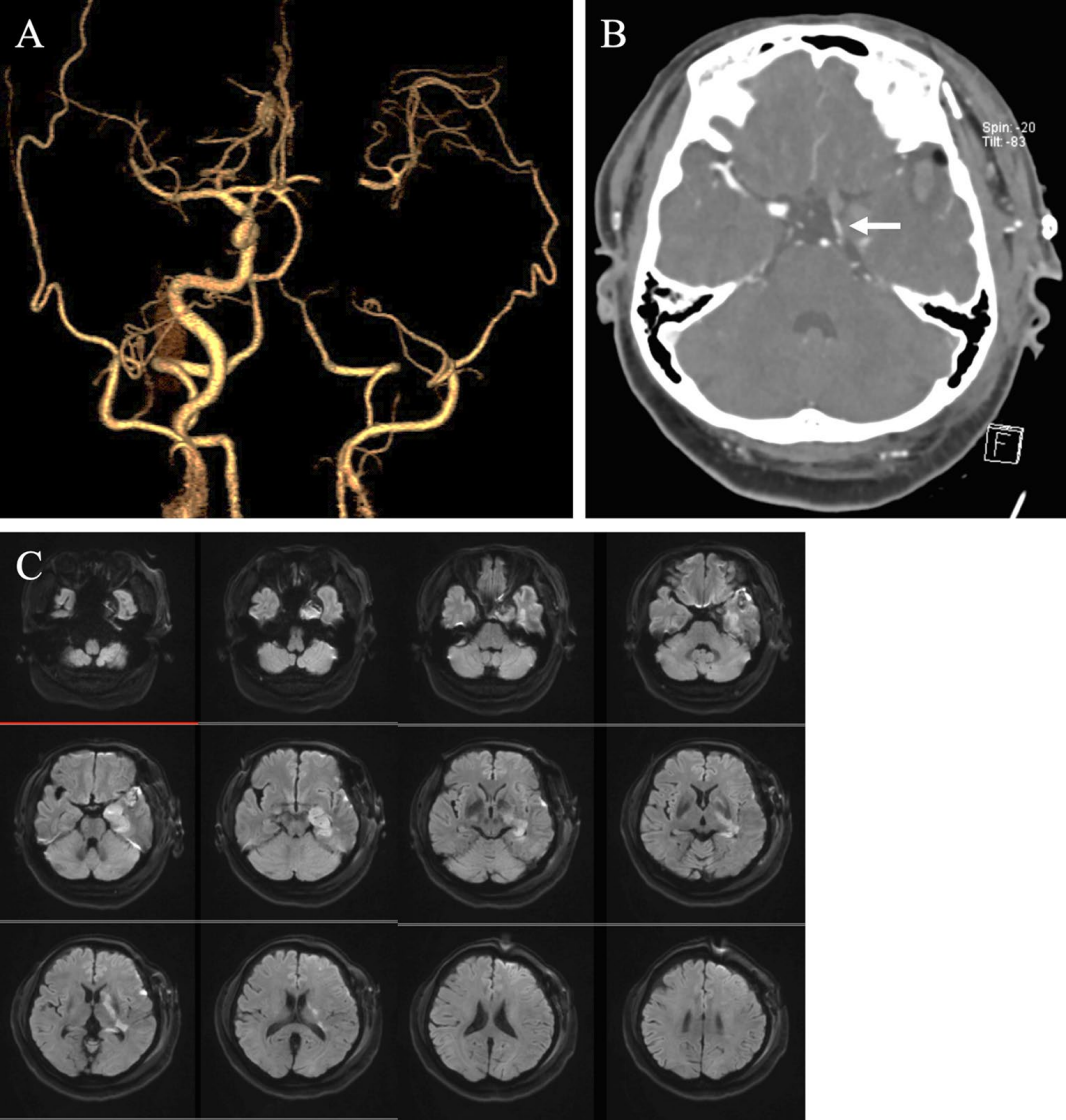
Postoperative computed tomography angiography and magnetic resonance imaging. (a) Double bypass flow was observed, although thrombus formation occurred until the left internal carotid artery terminus. (b) The left posterior communicating artery flow was confirmed (white arrow). (c) Cerebral infarction is observed in the anterior choroidal artery territory.
